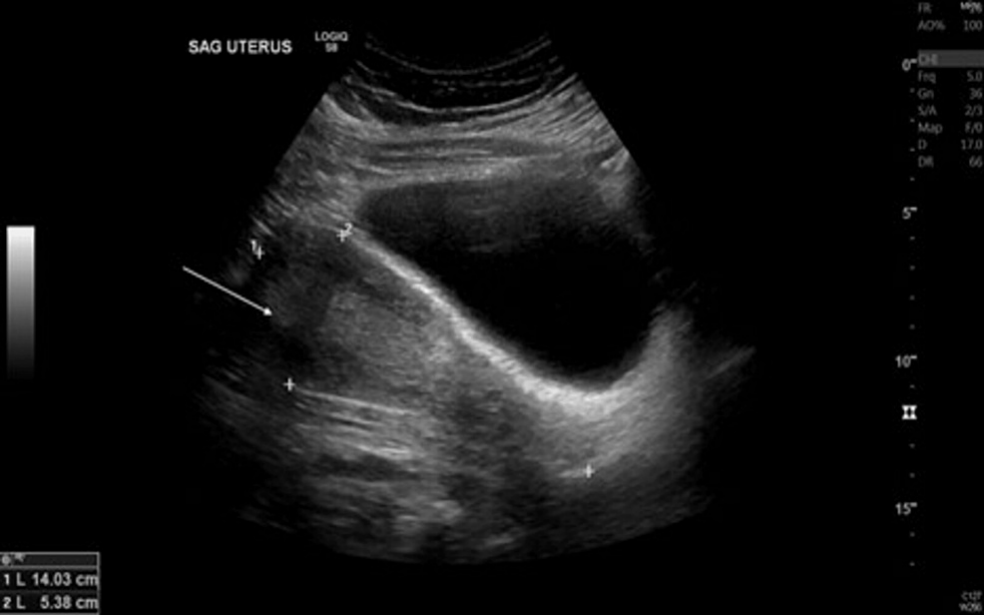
Transvaginal sonogram Arrow denotes ovarian mass; a large dark form is a uterus.
